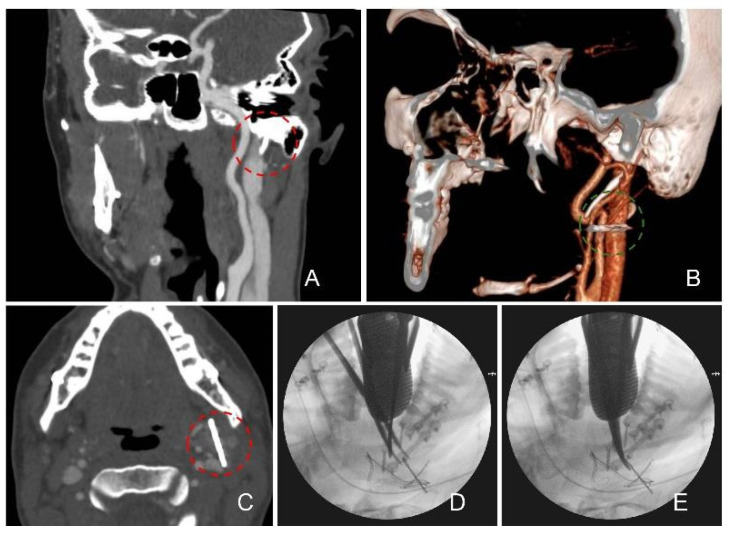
Coronal, 3D and axial Angio CT preoperative images localizing the foreign body in proximity of the right jugular vein (A–C); foreign body removal with intraoperative C-arm X-ray (D,E). The foreign body is indicated by a dotted circle.
